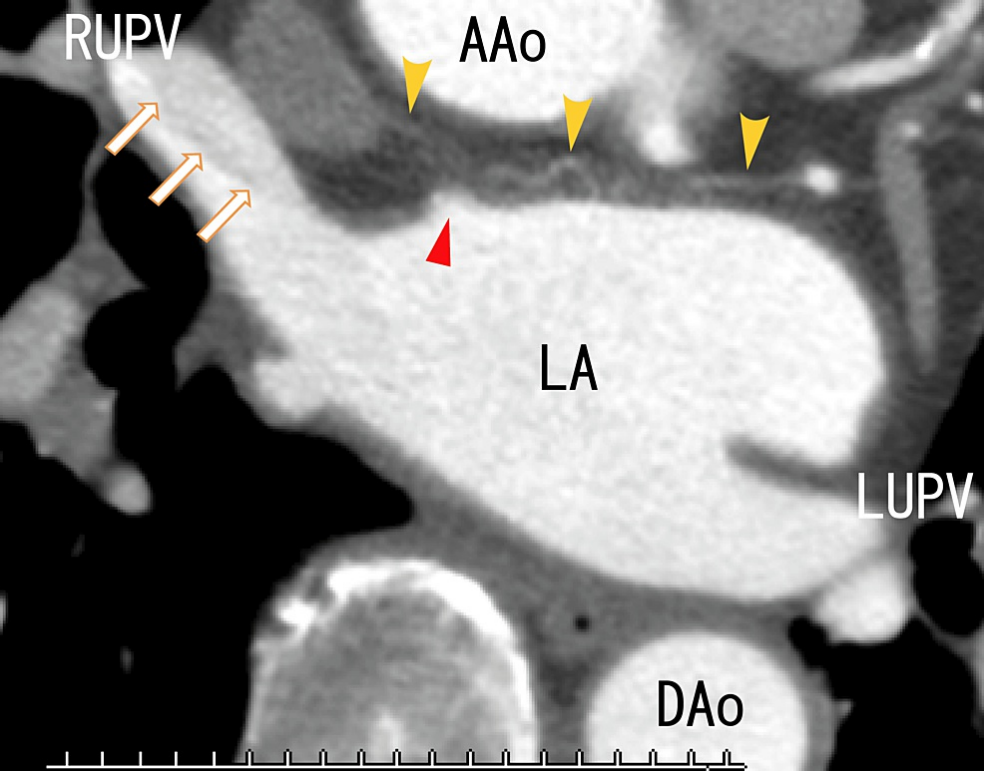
Axial 80-slice multidetector computed tomography (80-MDCT) images Axial 80-MDCT images show thrombi with clear margins in the RUPV (arrows). The LAD can be identified (red arrowhead). Collateral to the right atrium from the left circumflex artery (LCX) can vaguely be detected over the top of the LAD (yellow arrowheads). AAo: ascending aorta; DAo: descending aorta; LA: left atrium; LUPV: left upper pulmonary vein; RUPV: right upper pulmonary vein
